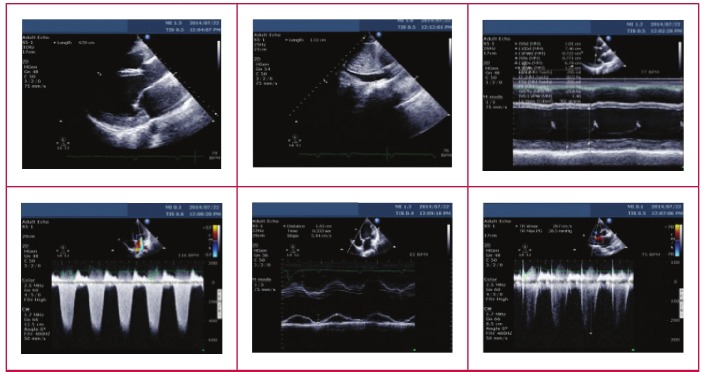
Echocardiography images depicting method of echo assessment in the study.
